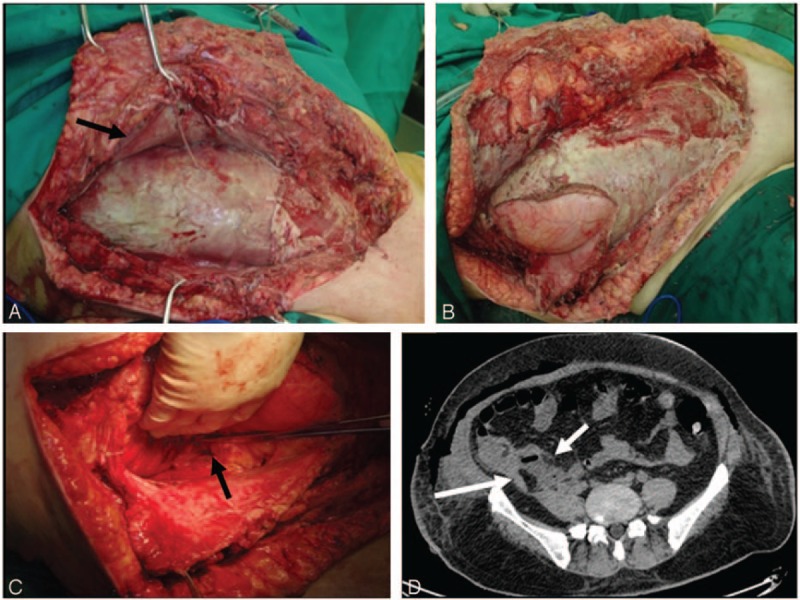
(A) The obliquus externus abdominis (arrow) was cut, and the aponeurosis of obliquus internus abdominis was infected. (B) The obliquus internus abdominis and transversus abdominis were cut. (C) A sinus tract (arrow) was traced which directly extended to the retroperitoneum. (D) Axial CT scan revealed a retroperitoneal abscess (short arrow), measuring 6.5 cm × 3.3 cm × 3.7 cm (vertical × anteroposterior × transverse), and the appendix (long arrow) was intact with mild swelling. CT = computed tomography.
